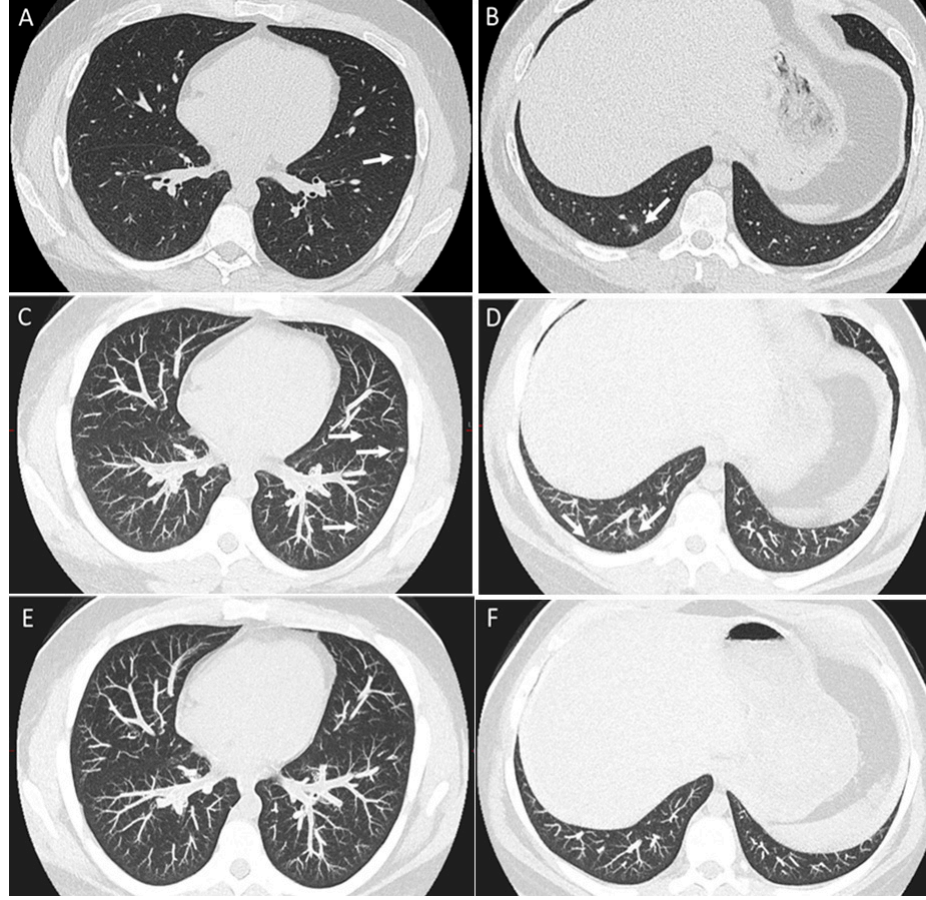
Patient with osteosarcoma and indeterminate pulmonary nodules. Axial non-enhanced images (A,B) and corresponding maximum-intensity projection images (C,D) show newly developed pulmonary nodules (arrows) for which short-term follow-up was recommended. Axial non-enhanced images using maximum-intensity projection (E,F) from a follow-up CT after three weeks show interval resolution of these nodules, which are compatible with resolved infection/inflammatory process.
